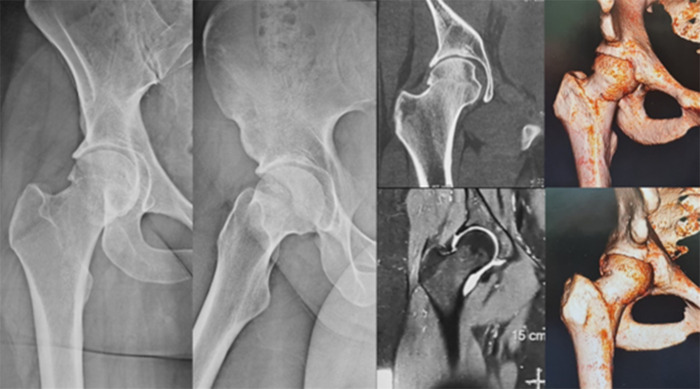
The full imaging profile needed for proper decision-making of a 28-year-old female presenting with right hip pain for two years. Plain weight-bearing radiographs (right), CT (upper left) and MRA (bottom left) reveal combined FAI with DJD.
